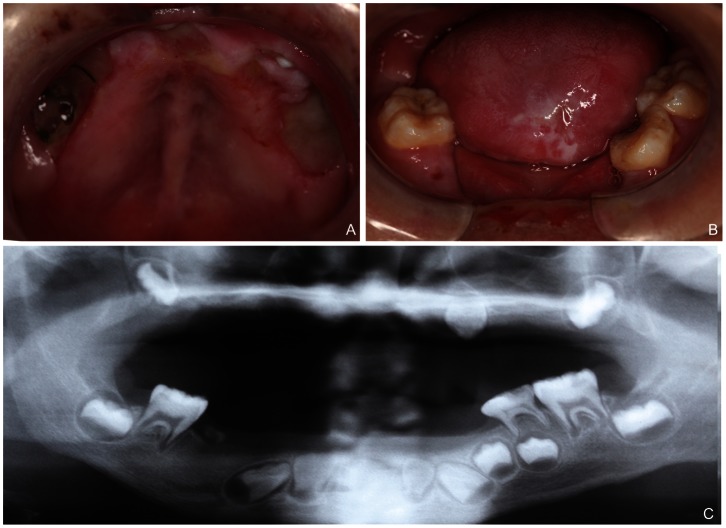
Oral manifestations of the proband. (A, B) Early teeth loss, the laceration and ulceration of oral soft tissues, dental attrition and dental caries. (C) Panoramic radiographs showing alveolar bone loss of the maxilla and mandible, exfoliation of 15 permanent teeth, and short root anomaly of the existing teeth.
